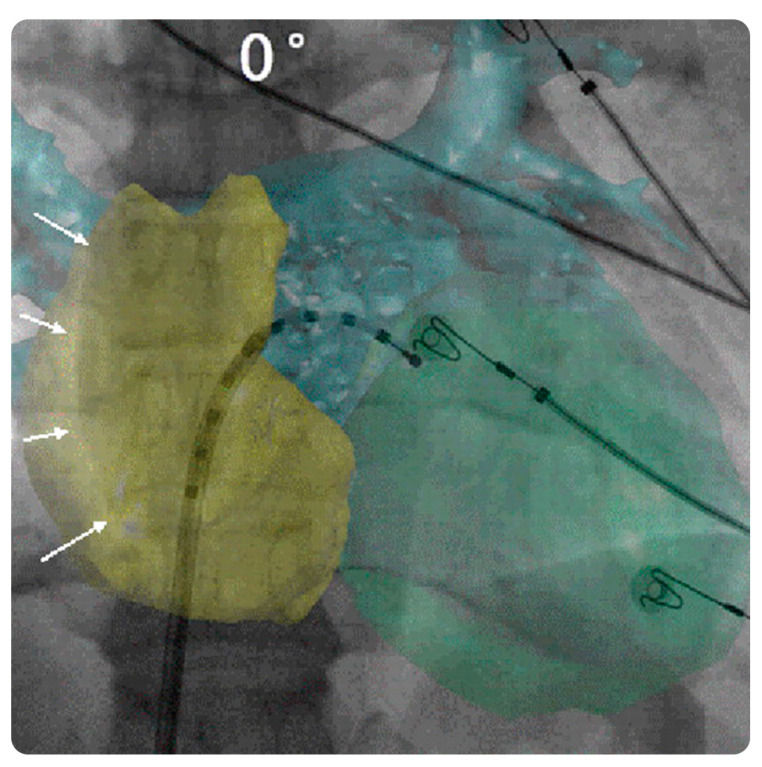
EPNavigator software and its role in intuitive display. CT registration based on heart contours (including reconstruction of both the atria and left ventricle). Antero-posterior fluoroscopic view with CT overlay. A decapolar catheter is inserted into the left atrium through a trans-septal sheath. The CT volume overlay is first aligned using the right lateral atrial contour (white arrows). Abbreviations: CT, computed tomography. Reprinted from “Computed tomography–fluoroscopy overlay evaluation during catheter ablation of left atrial arrhythmia” by Knecht et al. [66] with written approval from the corresponding author and publisher (EP Europace; Copyright 2008).
